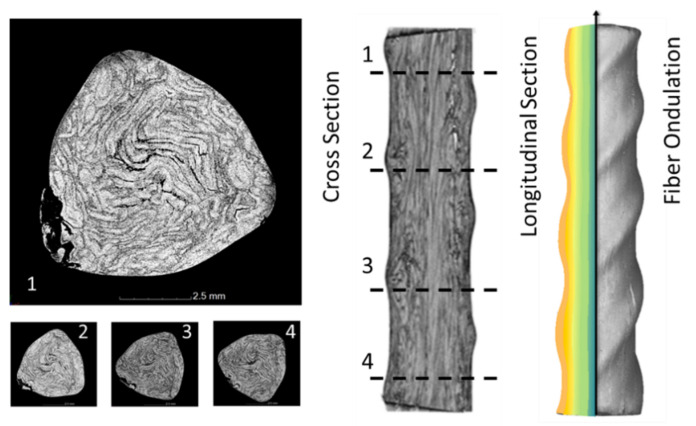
Computed tomography pictures to evaluate the axial and transverse fibre orientation in helix rebars.
